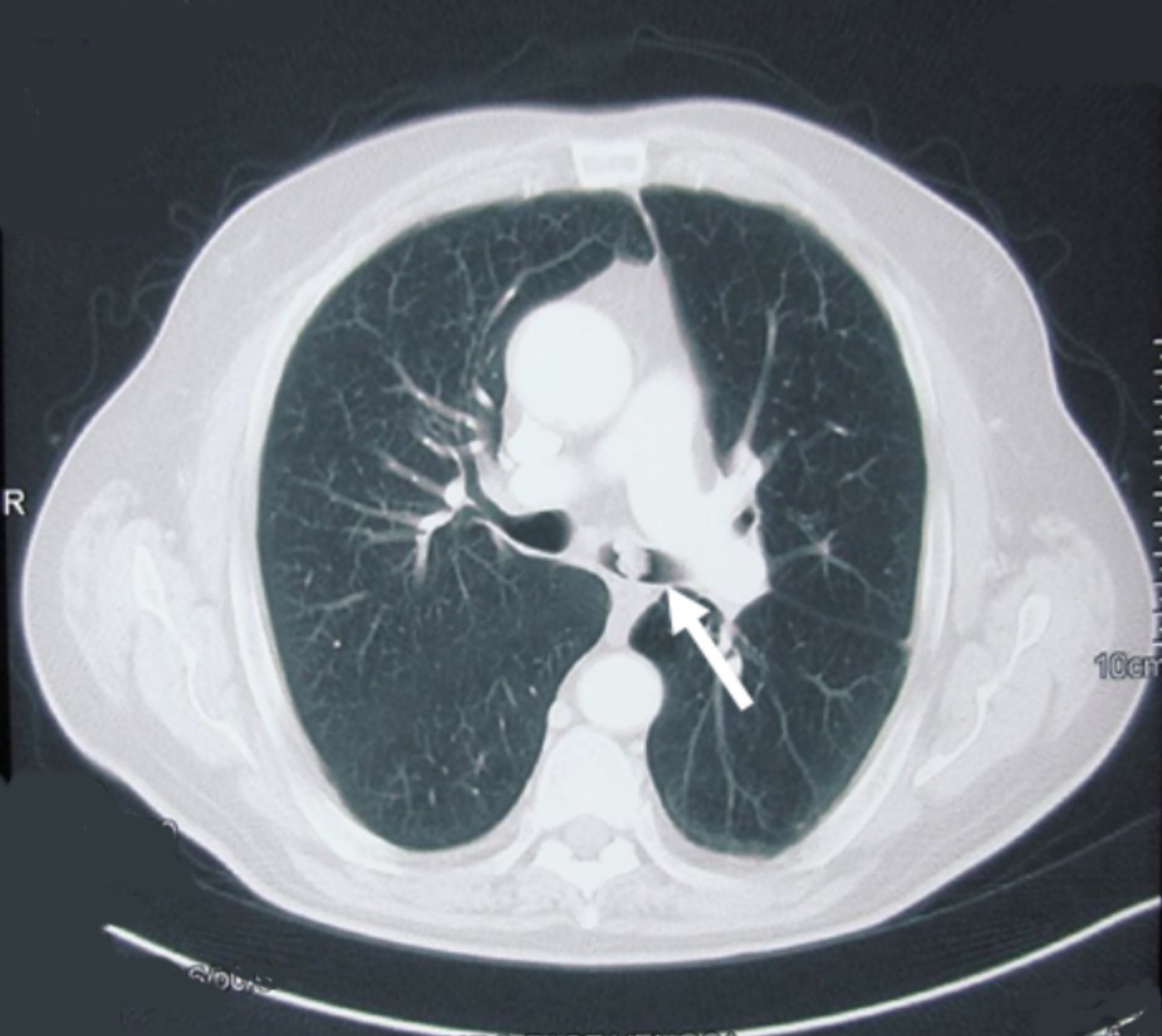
CT scan demonstrating a vegetating neoplasm of the left main bronchus (white arrow) without signs of extrabronchial infiltration.
